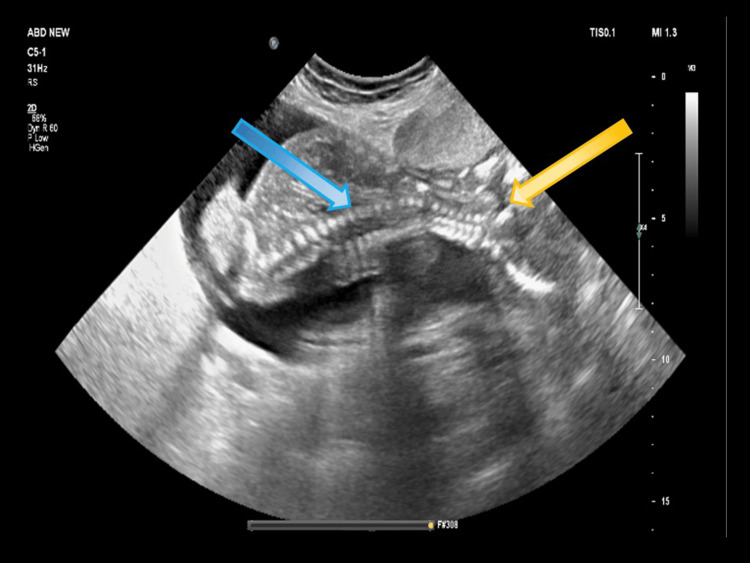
Grayscale ultrasonography image showing uterine rupture (yellow arrow) with spine of the fetus in the peritoneum (blue arrow).
